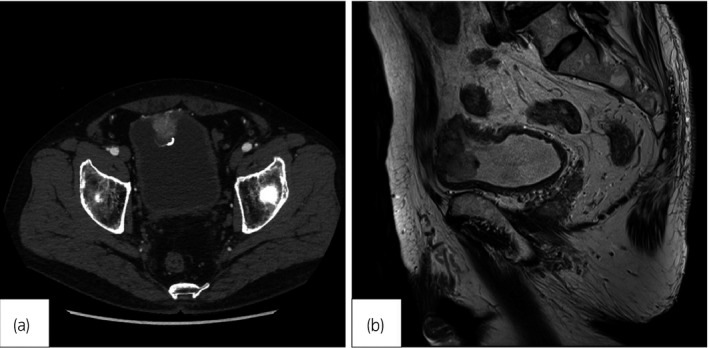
(a) CT revealed a 32 × 30‐mm bladder tumor covered with calcification at the top of the bladder. (b) MRI revealed a low intensity signal on T2‐WI, which suggested muscle‐invasive bladder tumor.
